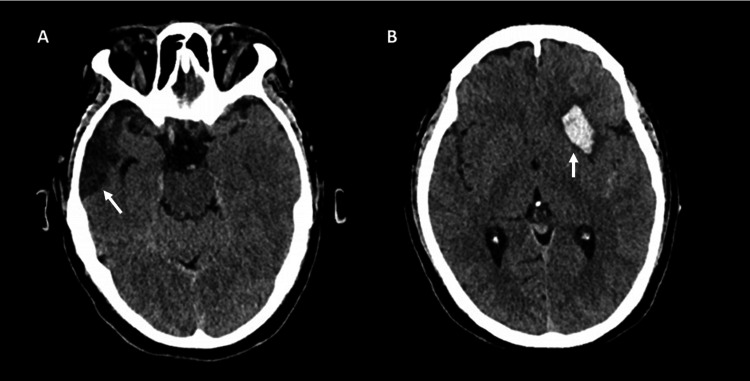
Non-contrast cranial CT scan. A: Encephalomalacia is identified in the right temporal pole (white arrow). B: An intraparenchymal left lenticular hematoma is demonstrated (white arrow).
